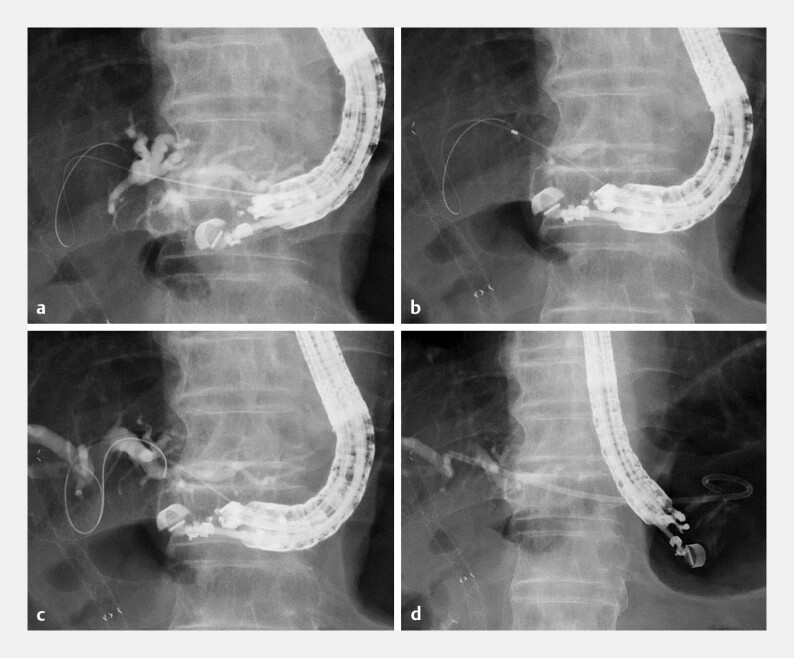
Endoscopic ultrasound-guided hepaticogastrostomy.
